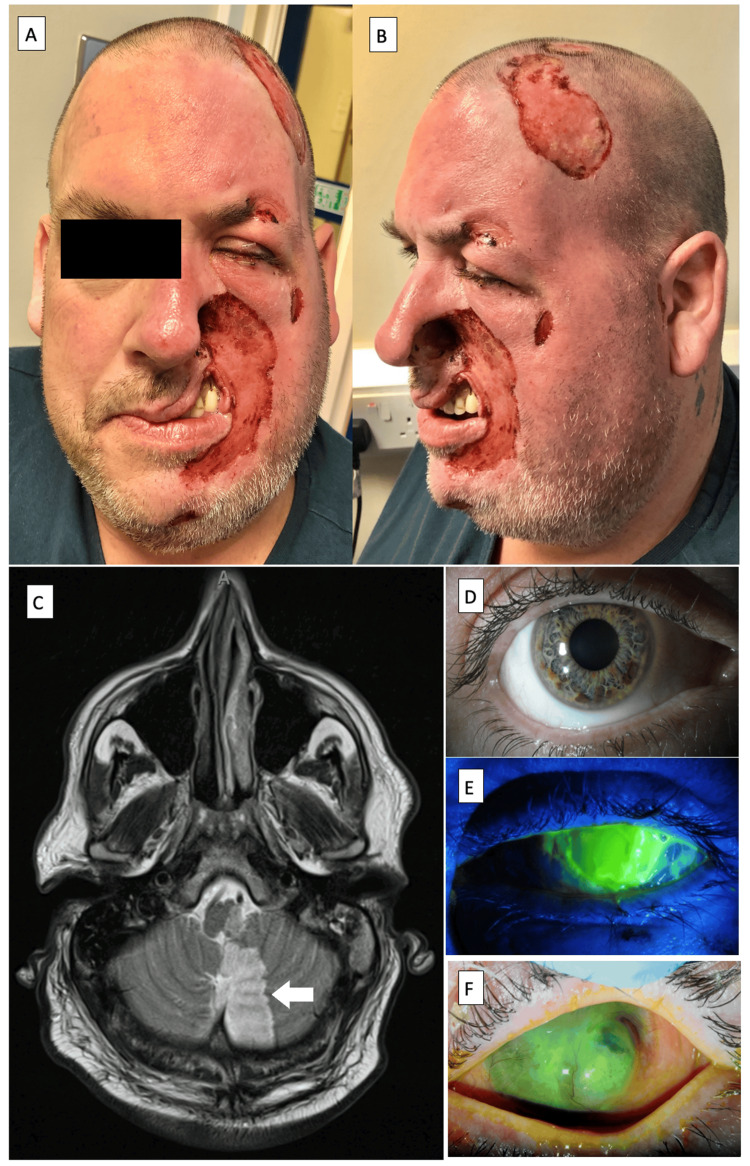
Images of case 1 (A,B) Clinical appearance at presentation with unilateral ulcers in the trigeminal territory, crescent-shaped, with ala nasi involvement, and sparing of the tip of the nose. (C) MRI T2/FLAIR sequence showed a large left posteroinferior cerebellar artery (PICA) territory infarct, affecting the inferomedial left cerebellar hemisphere and left side of the medulla. (D) Right eye showed unremarkable findings. (E) Left pancorneal epithelial defect, stromal thinning, and anesthesia. (F) Two months after treatment with topical antibiotics, an amniotic membrane graft, and a temporary tarsorrhaphy, the epithelial defect had completely healed, but the cornea remained opaque with superotemporal descematocele.
